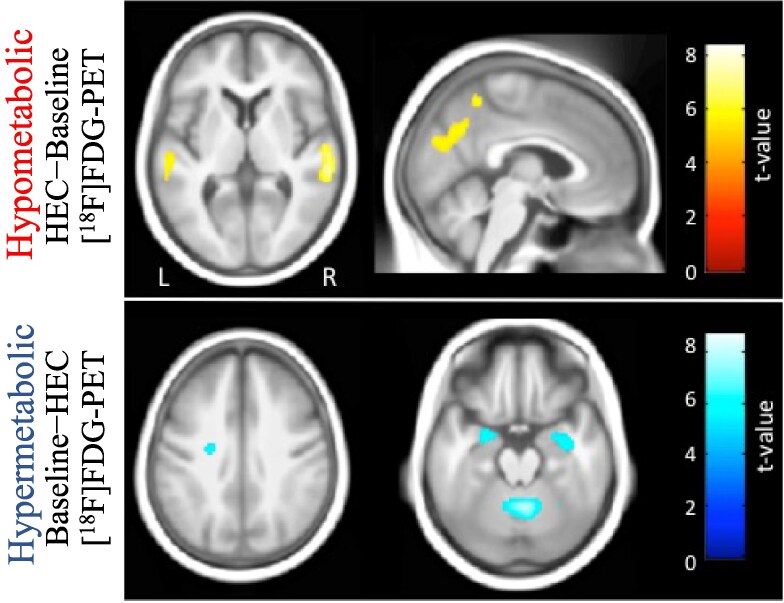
Example of hypo- and hypermetabolic regions. Representative sections showing the clamp effect with results overlaid over a mean structural image, the colour scale represents the corresponding *t*-value and images are displayed in neurological convention. HEC, hyperinsulinaemic euglycaemic clamp; [^18^F]FDG-PET, ^18^F-fluorodeoxyglucose positron emission tomography; L, left side; R, right side.
